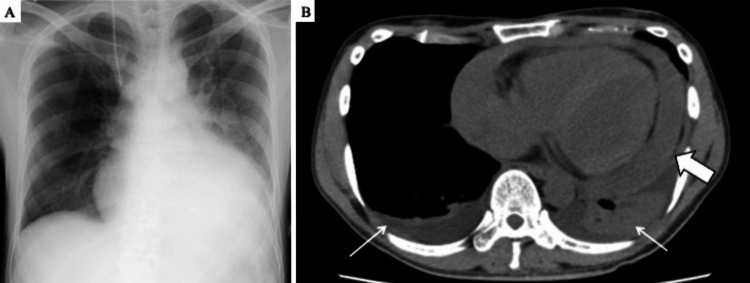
Imaging after five days of admission. (A) Chest radiograph showing an enlarged cardiac shadow with a cardiothoracic ratio of 66%. (B) Chest CT (axial view) showing pericardial effusion (arrow) and bilateral pleural effusions (narrow arrow).
